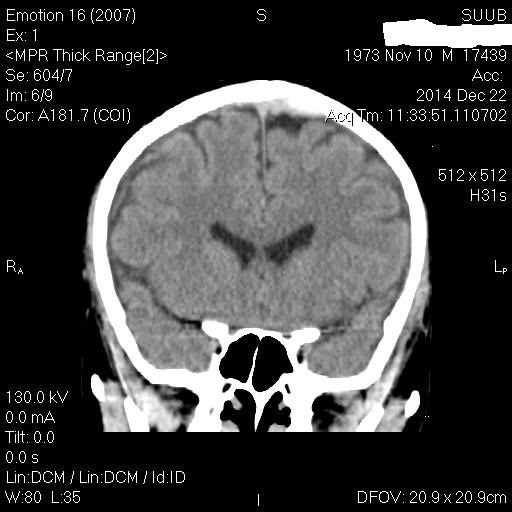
CT scan (coronal plane reconstruction) which evidences the high left frontal serohematic effusion
